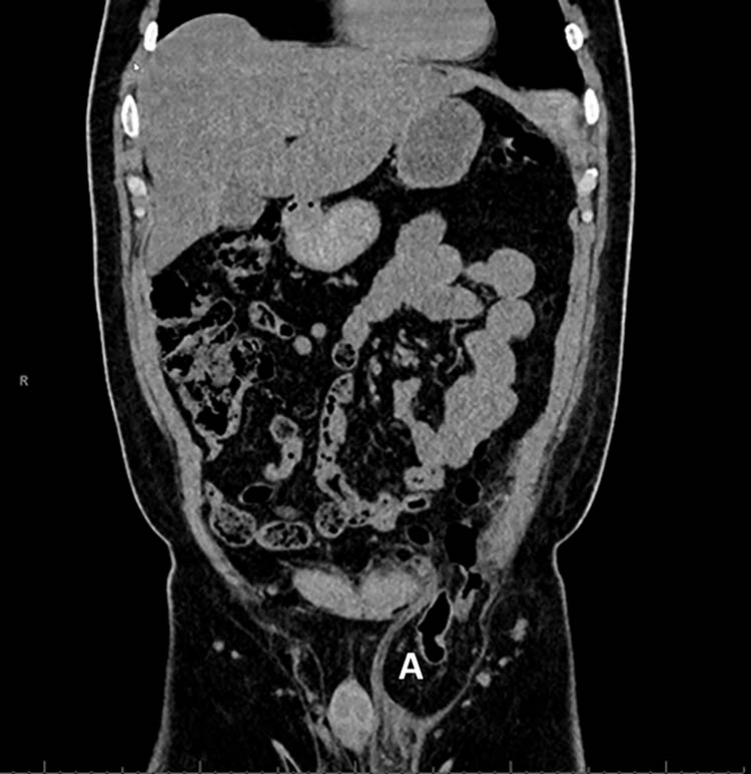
Computed tomography with sigmoid colon and solid tissue in hernia sac (A).
